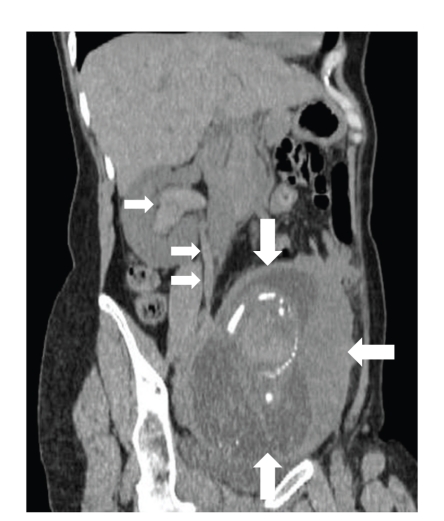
Longitudinal CT image indicating hematoma extending to the lower portion of the right ureter (small arrows) and a fetus in the uterus (large arrows).
